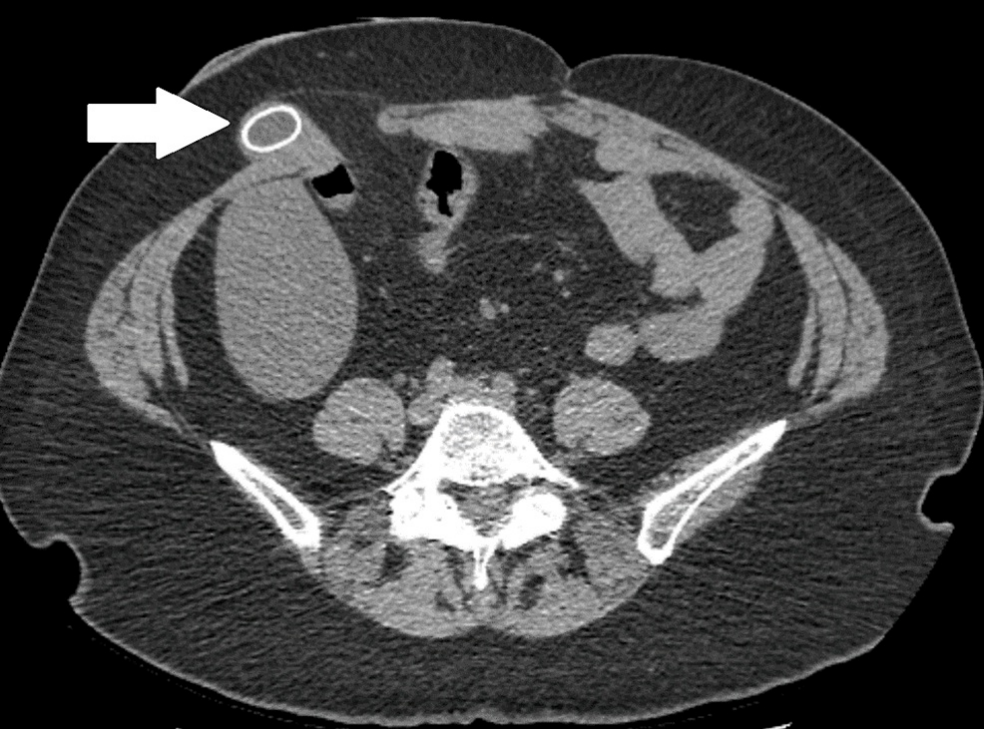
CT Abdomen and Pelvis with axial view of gallbladder herniation into parastomal hernia White arrow demonstrating gallbladder with 2-cm gallstone herniating through parastomal hernia
